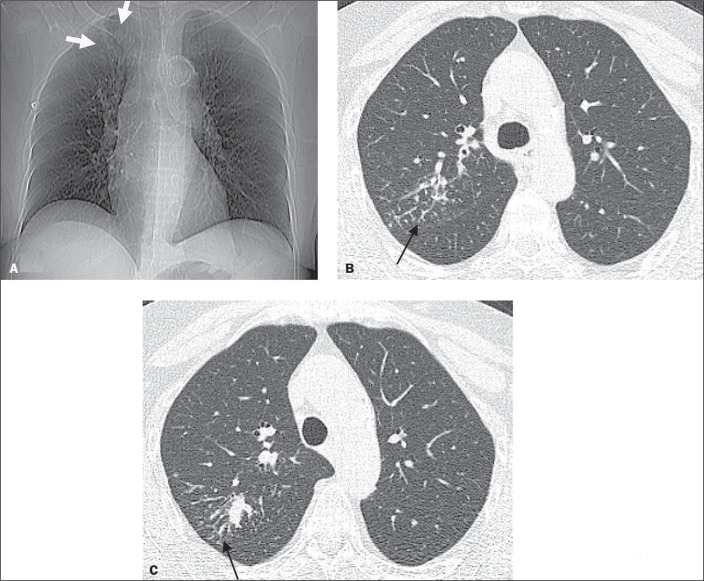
Chest X-ray (A) and HRCT (B,C) of a 58-year-old female with pulmonary TB (patient
